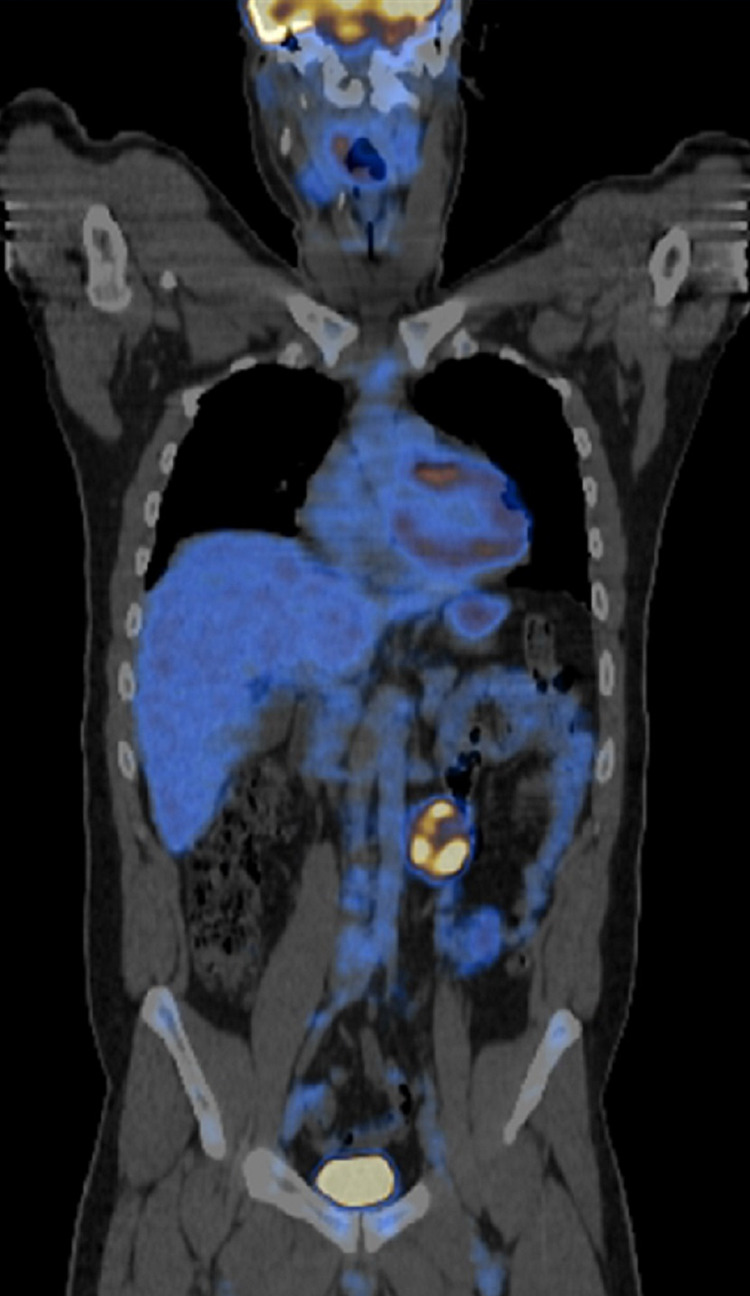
PET-FDG showing a left lateroaortic mass with associated glycolytic hypermetabolism in a coronal plane
